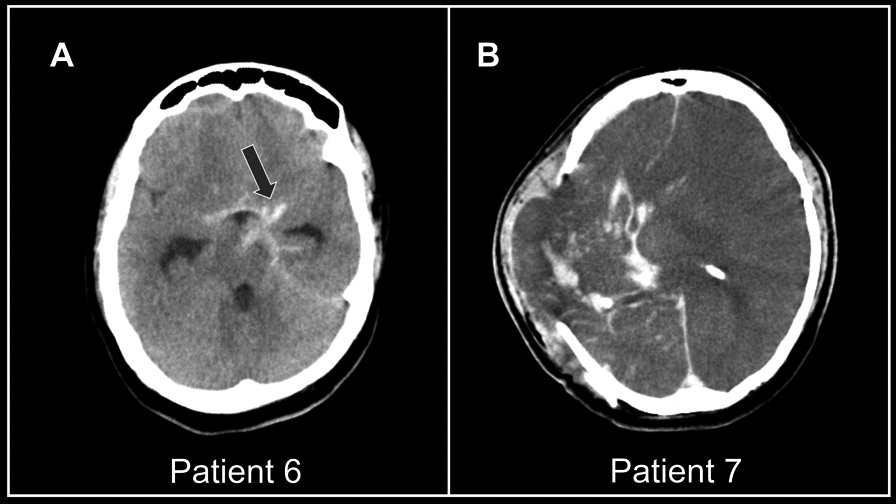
Brain imaging of patients 6 and 7. A Non-contrast CT image of the brain, as described for Patient 6, demonstrating subarachnoid haemorrhage (*arrow*) and acute hydrocephalus. B The postoperative non-contrast CT described in Patient 7 revealed right craniectomy, gross cerebral oedema, and right hemispherical herniation with intraparenchymal bleeding
